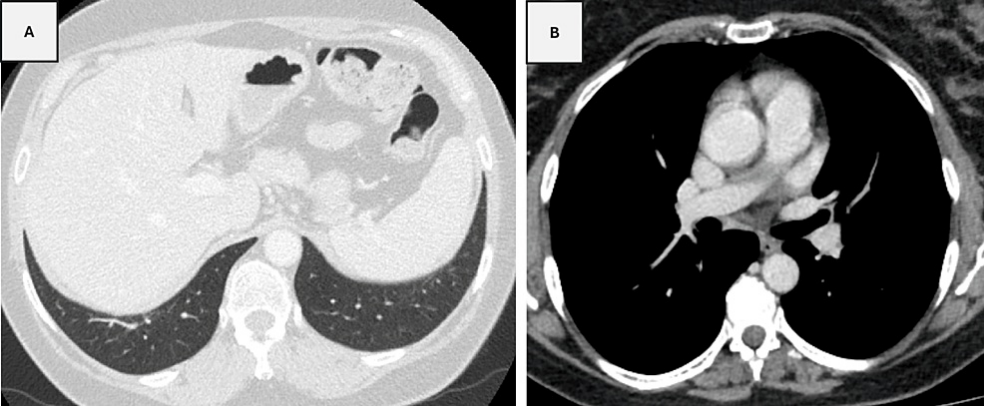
CT imaging showed resolution of the lung opacities (A) and lymphadenopathies (B).
